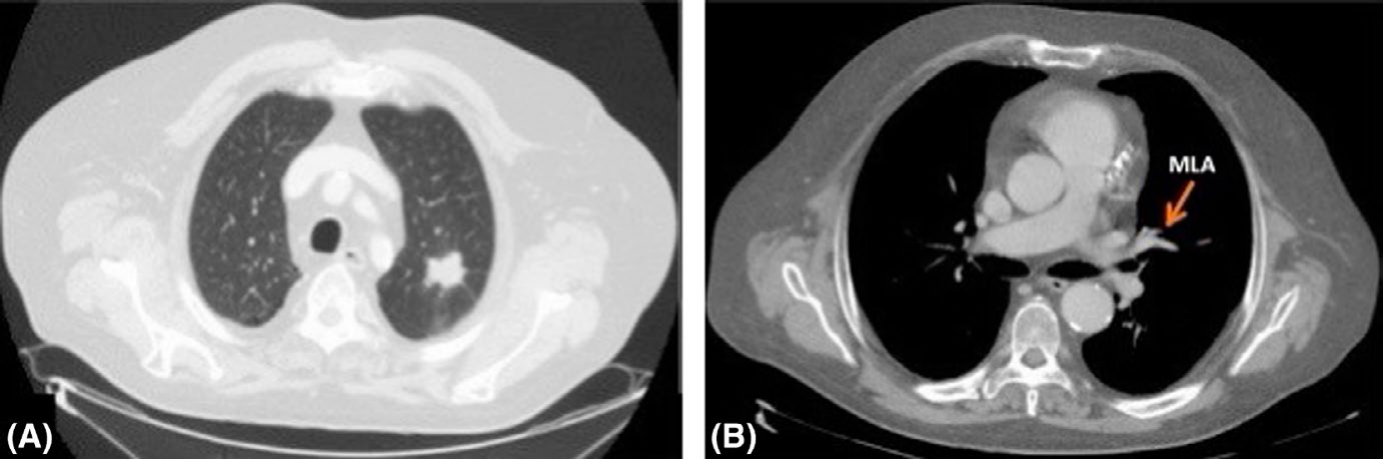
A, CT of the thorax with parenchymal window setting showing the left upper lobe lung cancer. B, Chest CT scan, viewed using mediastinal window, shows the so‐called mediastinal lingular artery arising from the anterior aspect of the mediastinal portion of the left pulmonary artery. MLA, mediastinal lingular artery
